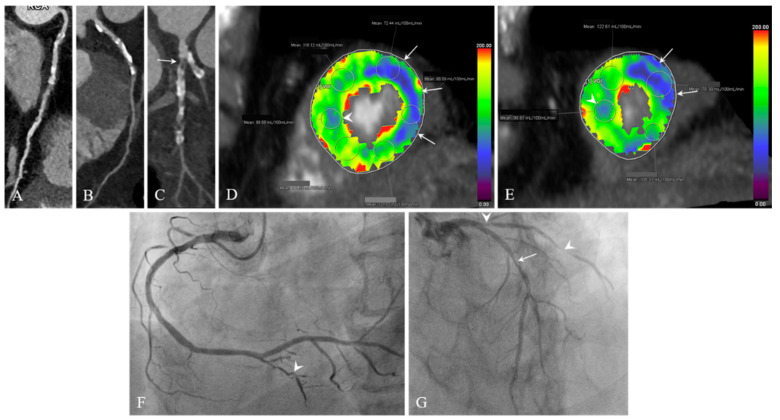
Images of a 74-year-old man with typical angina. (A–C) Curved MPR CCTA images of RCA (A), LAD (B), and LCx (C) show severe CAC. Severe stenosis with mixed calcified and noncalcified plaques is seen in the proximal portion of the LCx (C, arrow). (D,E) CT-MPIs show decreased MBF in the mid- to apical lateral (arrows), mid-anteroseptal (D, arrowhead), and apical septal (E, arrowhead) segments, with a greater decrease in the mid- to apical lateral segments. (F,G) ICA confirms significant stenosis (80% lumen reduction) in the small PDA (F, arrowhead) and proximal and distal LCxs (G, arrowheads), and significant stenosis (65% lumen reduction) in the proximal to middle LAD (G, arrow). The invasive FFR for the middle LAD is 0.70. CT-MPI = computed tomography-myocardial perfusion imaging, CCTA = coronary computed tomography angiography, MPR = multiplanar reformation, MBF = myocardial blood flow, ICA = invasive coronary angiography, FFR = fractional flow reserve, PDA = posterior descending artery, RCA = right coronary artery, LAD = left anterior descending coronary artery, LCx = left circumflex artery.
